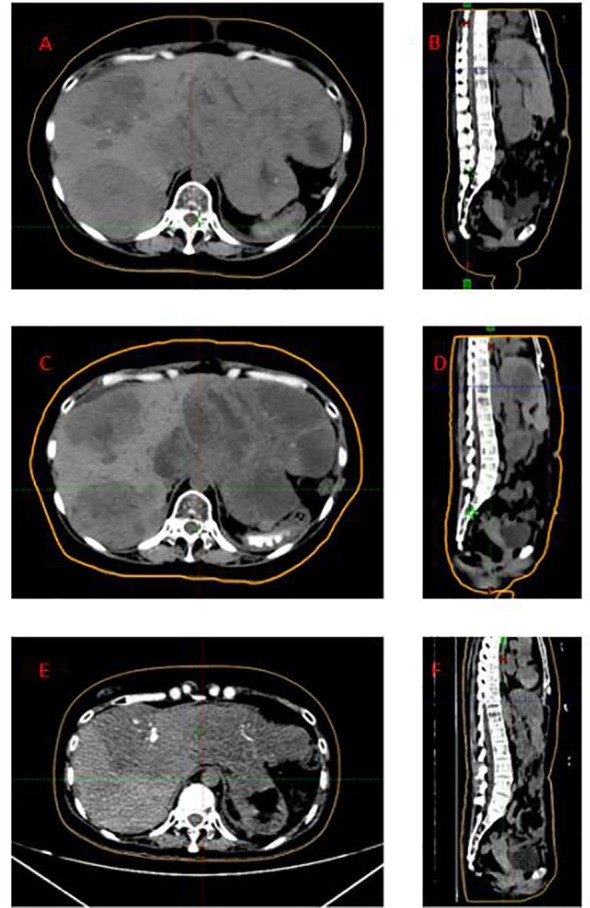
Sequential CT images demonstrating tumor response over time. (A, B) In April 17, 2025, the left lobe measuring up to 137 × 127 mm. (C, D) In May 20, 2025, the left hepatic lobe tumor to 121 × 97 mm. (E, F) In September 1, 2025, the left hepatic lobe mass to 110 × 87 mm, with slight shrinkage of other lesions and regression of hilar lymph nodes. The patient was in good general condition, without abdominal tenderness or lower limb edema.
